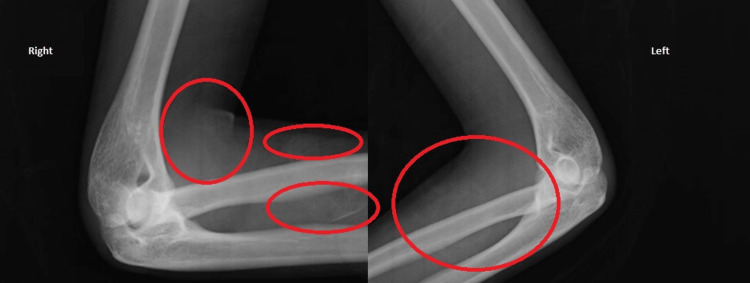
Elbow radiographs. Note the increased opacity of the peri-articular soft tissues, indicating the development of calcinosis.
